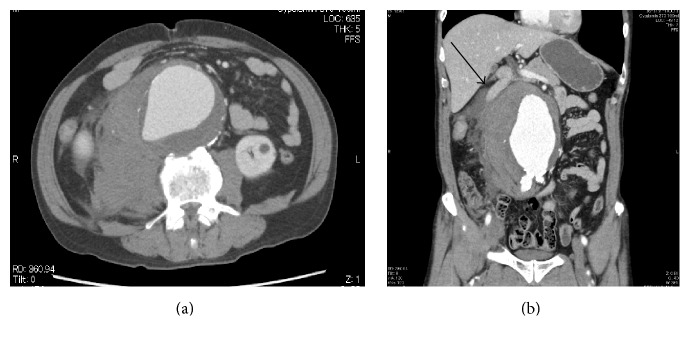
(a) Enhanced computed tomography revealing a ruptured aortic aneurysm measuring 7.9 × 7.6 cm in diameter in the right retroperitoneal region. (b) The inferior vena cava is compressed by a hematoma (black arrow).
